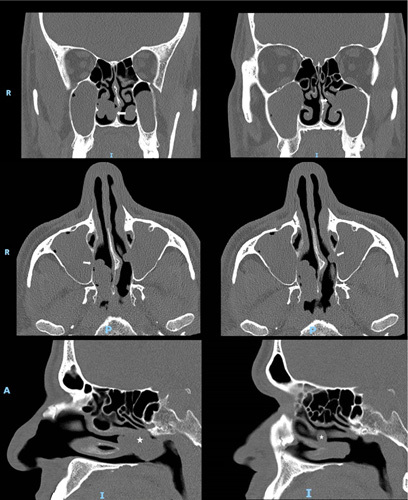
Computed tomography (CT) scan (coronal, axial, and sagittal views) of the paranasal sinuses depicting bilateral antrochoanal polyps (arrows, asterisks).
